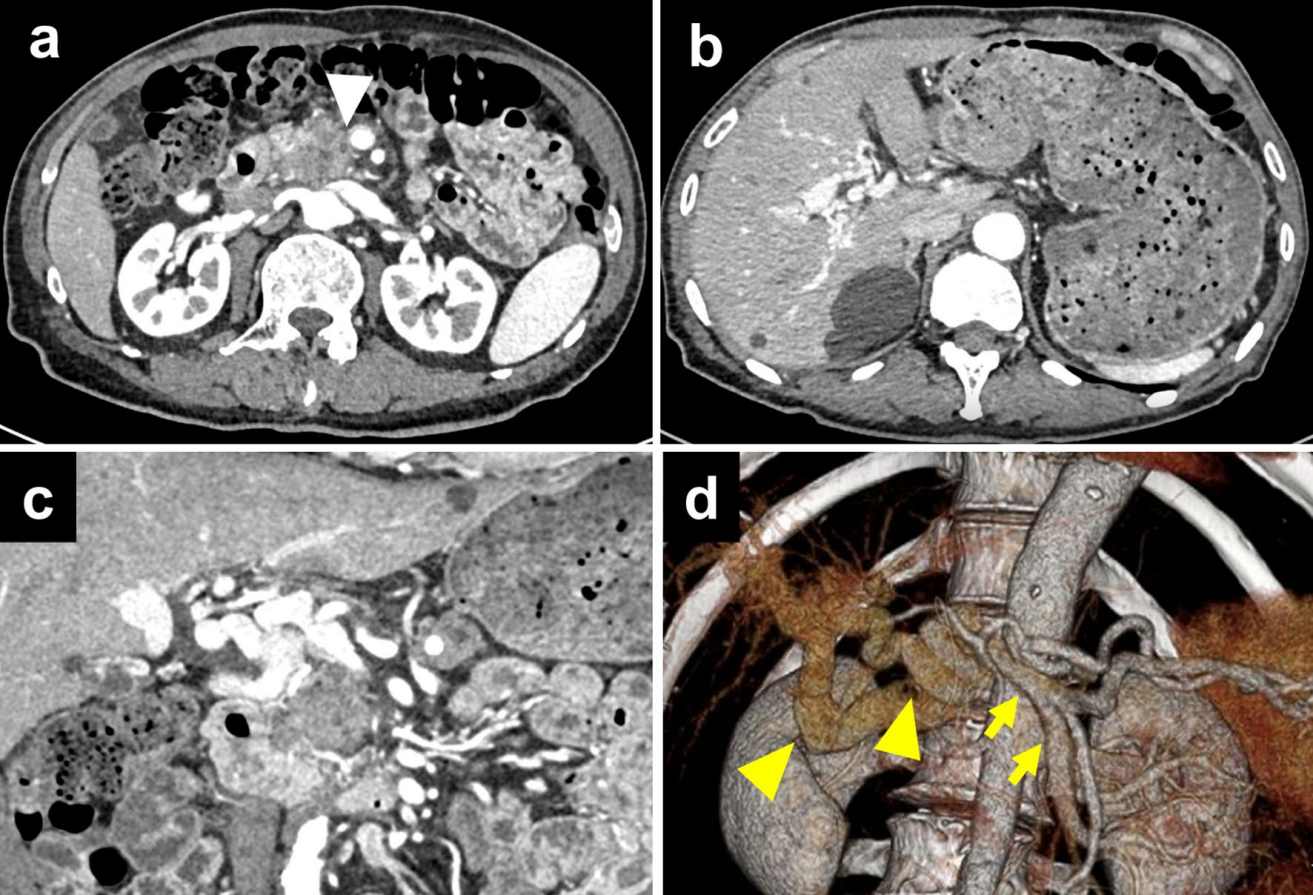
a An enhanced CT indicated a tumor abutted on the superior mesenteric vein in the level of maximum short-axis diameter (white arrowhead). b Axial view of the CT examination revealed cavernous transformation around the hepatic hilum. c Cranial view of the CT showed serpiginous collateral vessels leading to the intrahepatic portal vein. d Three-dimensional reconstruction image of the CT examination showed that two main collateral vessels (yellow arrowheads) existed around the hepatic portal region, and the superior mesenteric vein, the splenic vein and the portal vein in the pancreas had no sign of tumor invasion (yellow arrows)
